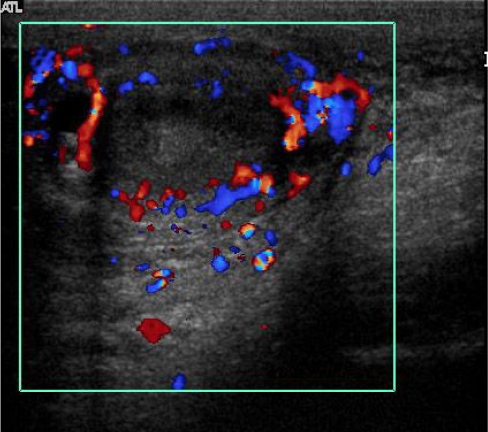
Colour Doppler US of the left testis shows increased peritesticular vascularity but absence of intravascularity indicating testicular ischemia.
